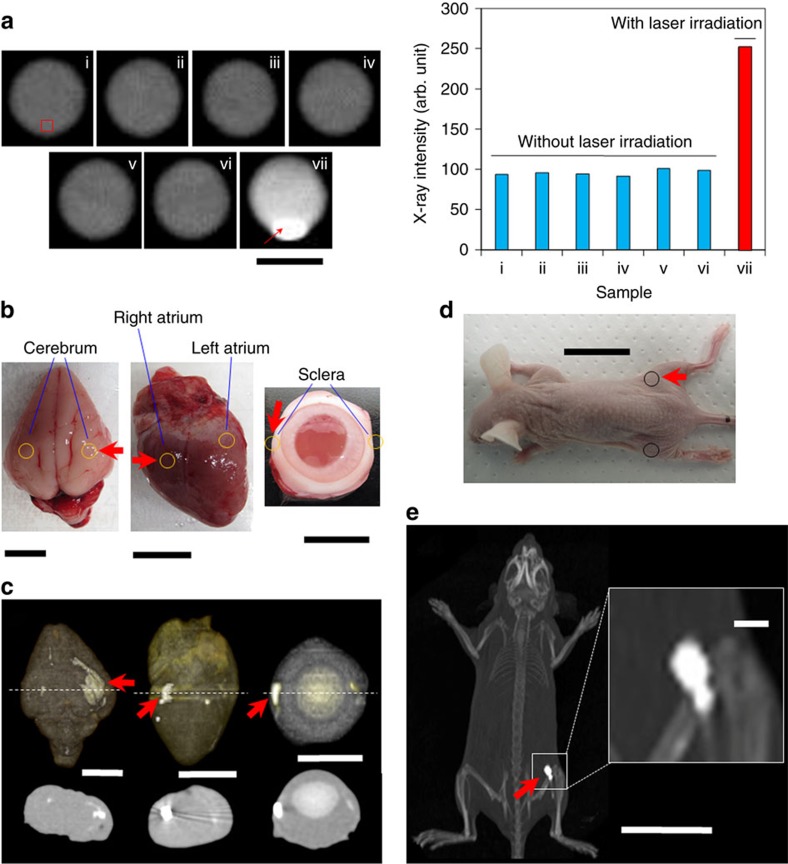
Targeting X-ray-enhanced imaging by laser-induced transformation of LM
